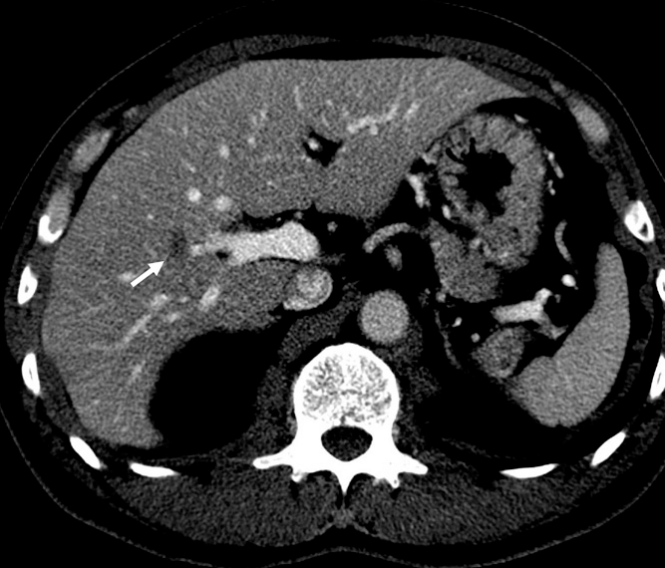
Contrast-enhanced CT shows a small, oval, low-attenuating nodule in the right lobe of the liver adjacent to the anterior branch of the right portal vein (arrow).
